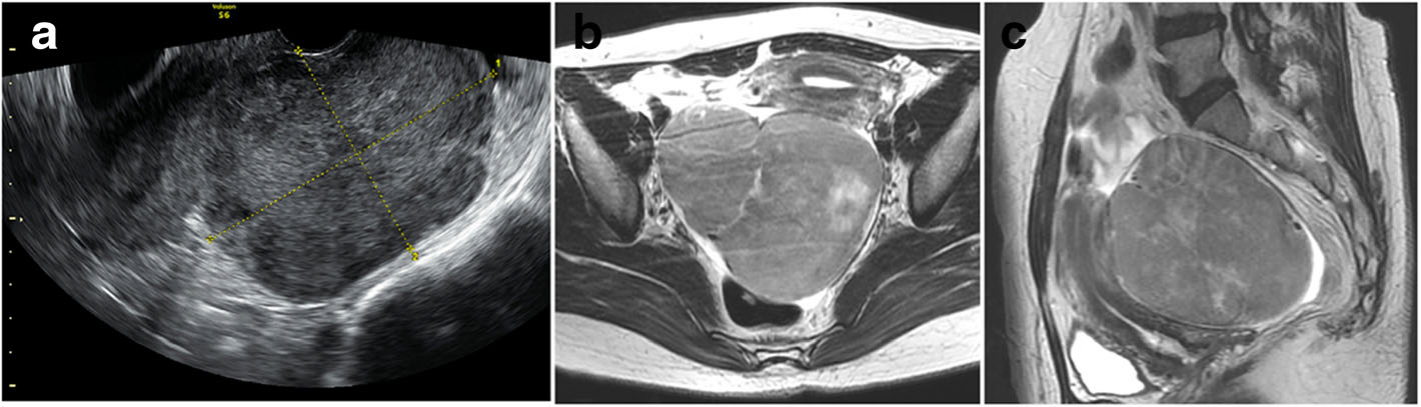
Preoperative transvaginal ultrasonography (a) detected an isoechoic solid mass in the right pelvic cavity (approximately 10 cm in size) with an ill-defined boundary between the lesion and the uterus, and T2-weighted magnetic resonance imaging of the ovarian tumor in (b) the horizontal and (c) the sagittal planes
